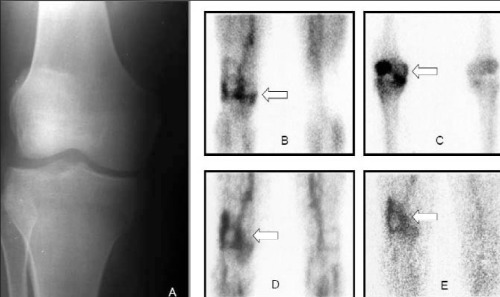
A 32-y-old male with the diagnosis of AS for 4 years. Thepatient was complaining of pain on the right knee. ESR and CRPwere found to be high but there was not active inflammation inclinical evaluation. Radiography (A), blood pool (B) and 4^th^ hour planarMDP (C) images, 4^th^ hour (D), 24^th^ hour HIG (E) images (bothanterior views). A marked hyperemia over the right knee joint andosteoblastic activity over patella, medial epicondyl, proximal tibia wasobserved on bone scan. HIG scan was similar to bone scan exceptthat the hyperemia and HIG accumulation on 4th hour images weremore prominent in lateral side of distal femur indicating a possibleinflammation of the tendon (arrows)
